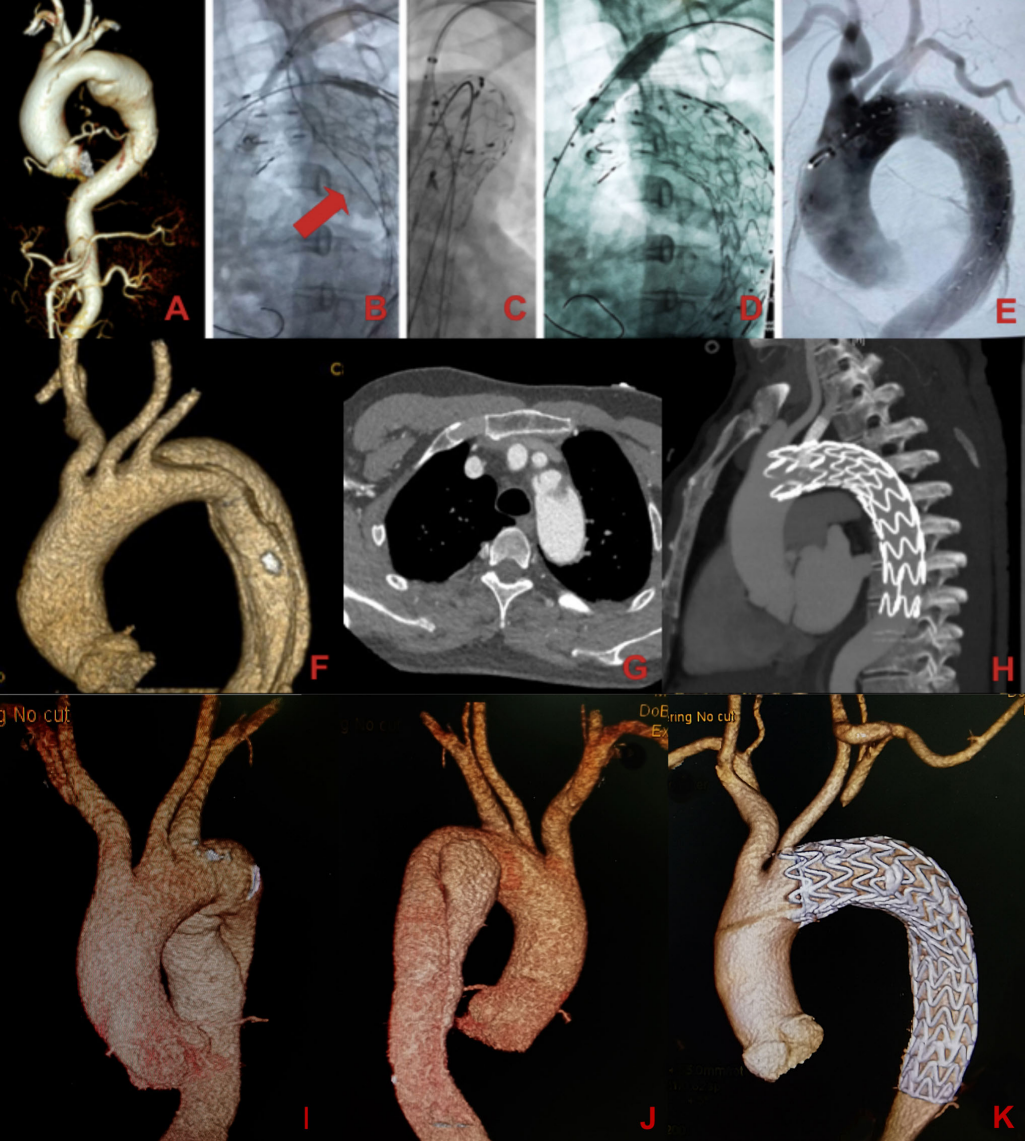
Cases in the study. A–E The binding stent-graft puncture system (BPS) in in situ needle fenestration (ISNF) to revascularize multiple aortic branches. A Preoperative CTA shows an aortic aneurysm with hostile landing zone for TEVAR. B The binding guidewire (red arrow) was advanced toward the abdominal aorta to bind the system firmly. C The thoracic stent-graft was punctured and the guidewire was advanced into the aortic lumen successfully. D A balloon-expandable branch stent was deployed with its proximal side in the aortic lumen, with its distal side in the branch. E The completion DSA shows ideal results. F, G, H Results after successful ISNF for a patient with aortic dissection. I, J, K Results after successful carotid-subclavian bypass with TEVAR for a patient with aortic dissection
